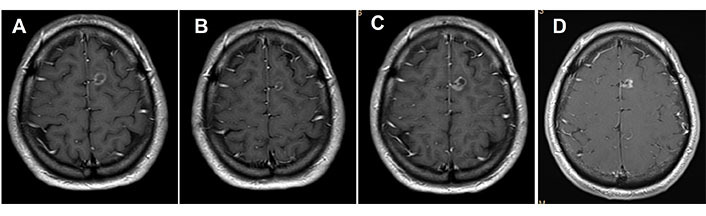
MRI of the brain. (A) Basal brain metastasis; (B) with partial response to osimertinib treatment; (C) progression after 14 cycles of osimertinib; (D) complete response after receiving SRS 20 Gy treatment
